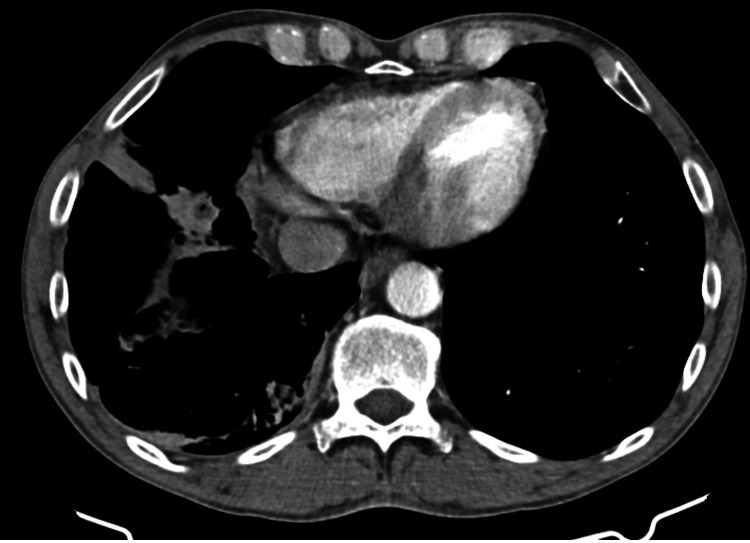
CT showing curvilinear hyper-density in the right lower lobe region
